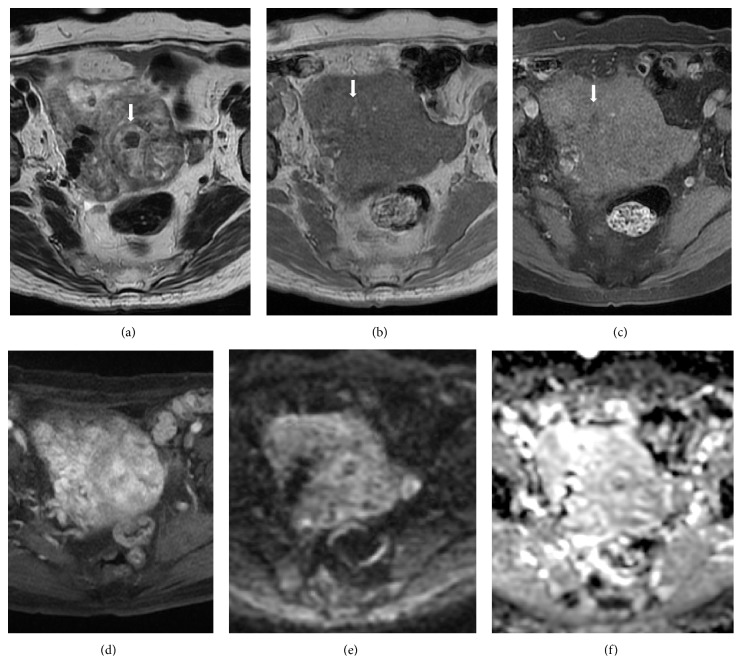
On MR images, the uterine tumor, approximately 13 × 7 × 6 cm in size, extensively involved the myometrium as a poorly demarcated diffuse lesion. (a) Axial T2-weighted image showed the lesion involving a few hypointense nodules (arrow) as having inhomogeneous high signal intensity (SI). (b) Axial T1-weighted image showed the lesion with a number of hyperintense tiny foci (arrow) as having low SI similar to that of the myometrium. (c) On the axial fat-suppressed T1-weighted image, the hyperintense foci within the lesion on T1-weighted images were suppressed (arrow), reflecting a lipomatous tumor. (d) On the contrast-enhanced fat-suppressed T1-weighted image, the lesion demonstrated strong contrast enhancement but contained several poorly demarcated hypointense areas, reflecting degenerative or necrotic changes. ((e), (f)) On diffusion-weighted images (e) and the ADC map (f), the lesion showed slightly high SI.
